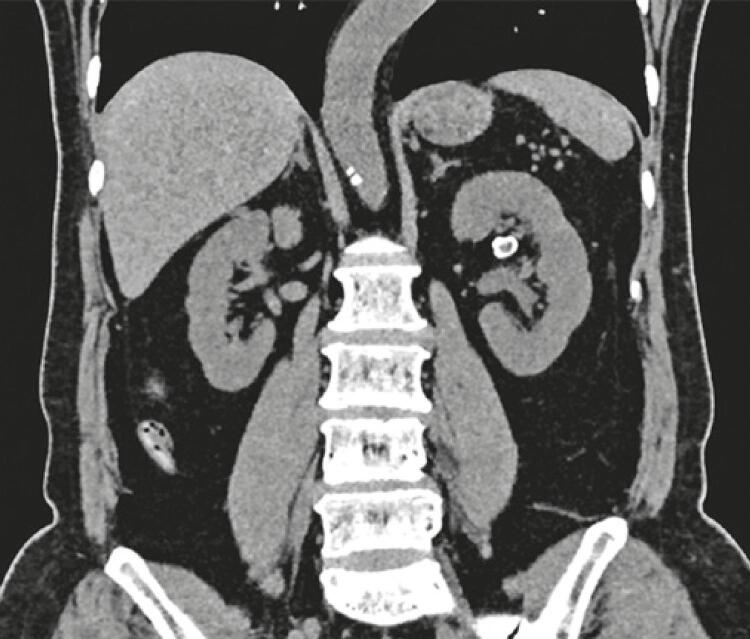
Computed tomography scan showing a saccular aneurysm with parietal calcification of the left renal artery on the plane of the renal hilum, measuring 1.0cm x 1.0cm (coronal plane)
